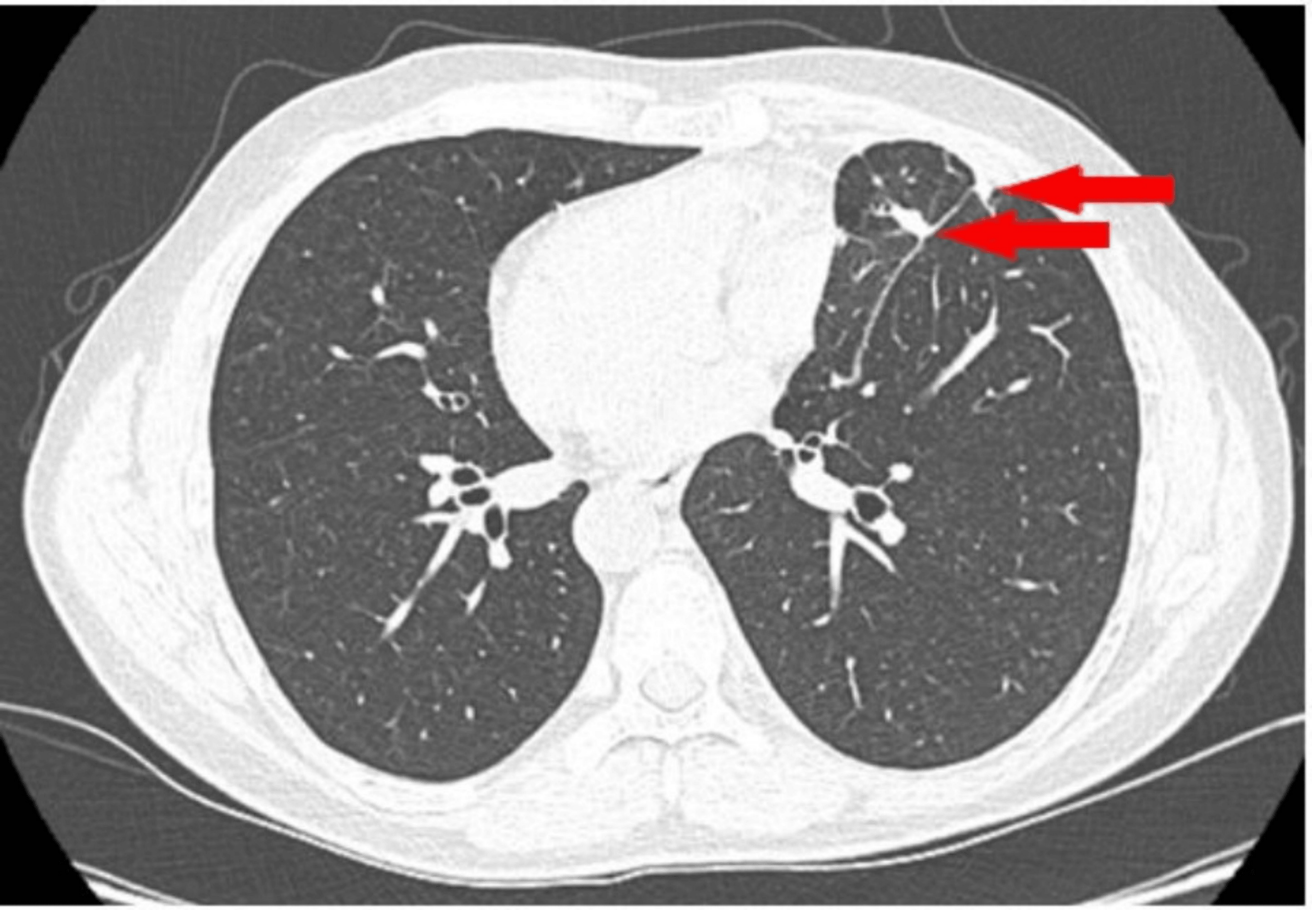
Cest CT postoperative residual changes
